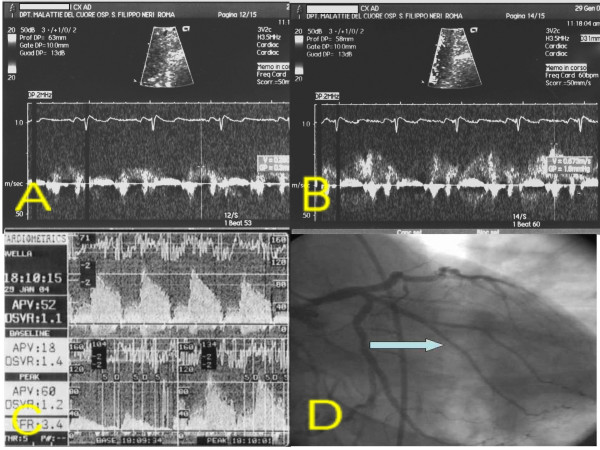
Patients 2. A: TTDE before adenosine. B: TTDE after adenosine. C: DW recording. D: coronary angiography with site of DW recording (arrow). DW: Doppler-wire. TTDE: transthoracic Doppler echocardiography.
